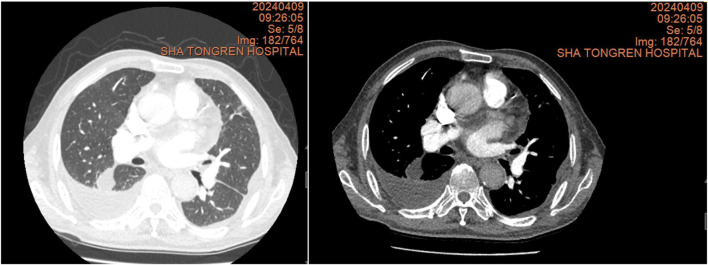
Chest CT scan from 9 April 2024, showing a significant increase in right pleural effusion compared to 28 February 2023, with a small amount of loculated effusion.
